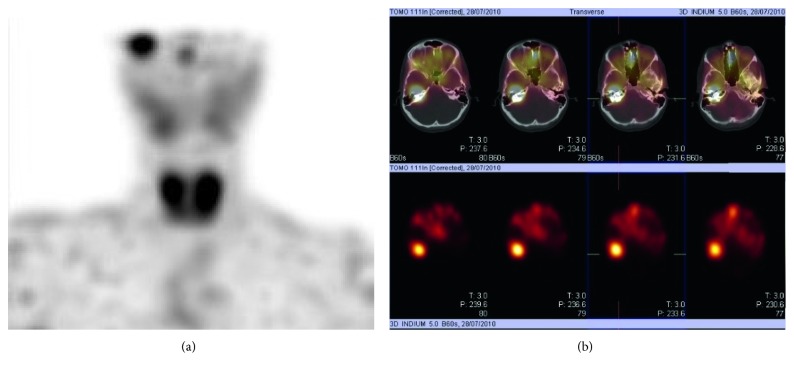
(a) ^111^In-pentetreotide scintigraphy from 2010 (MIP) intense activity in the right ME. (b) ^111^In-pentetreotide scintigraphy from 2010 (fused transverse SPECT/CT images and transverse SPECT images).
